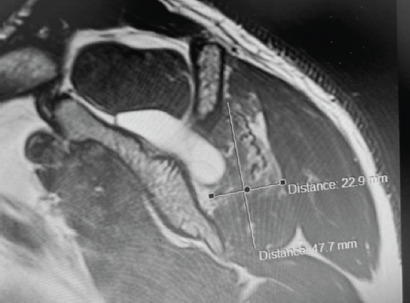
T2 weighted image showing a persistent spinoglenoid cyst of the shoulder following initial surgery. There is further atrophy of the infraspinatus muscle compared to the earlier MRI pre-operatively.
